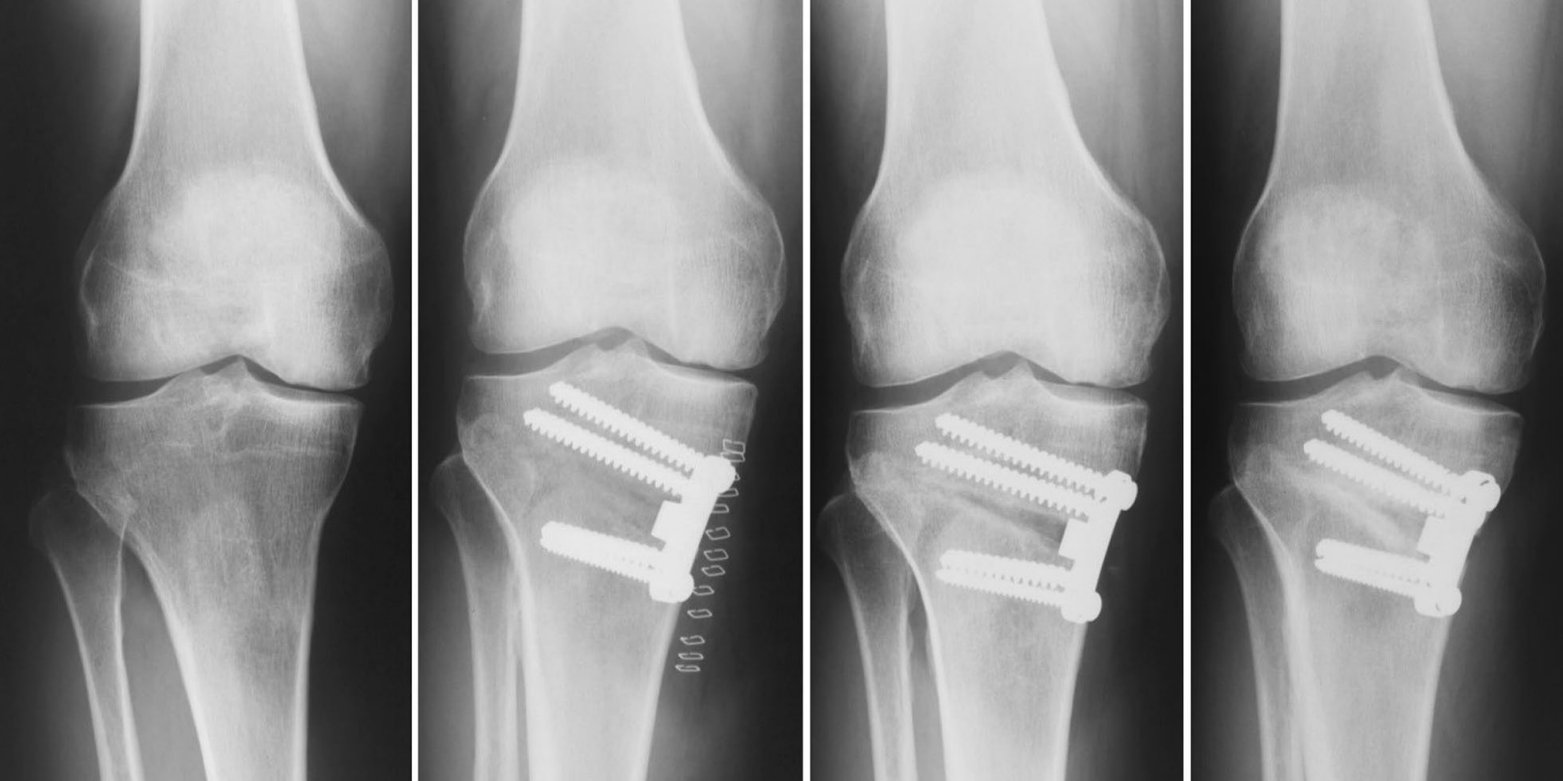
Anteroposterior X-ray views of the same right knee. From left to right, respectively: preoperatively, and 1 day, 1 month, and 3 months postoperatively
